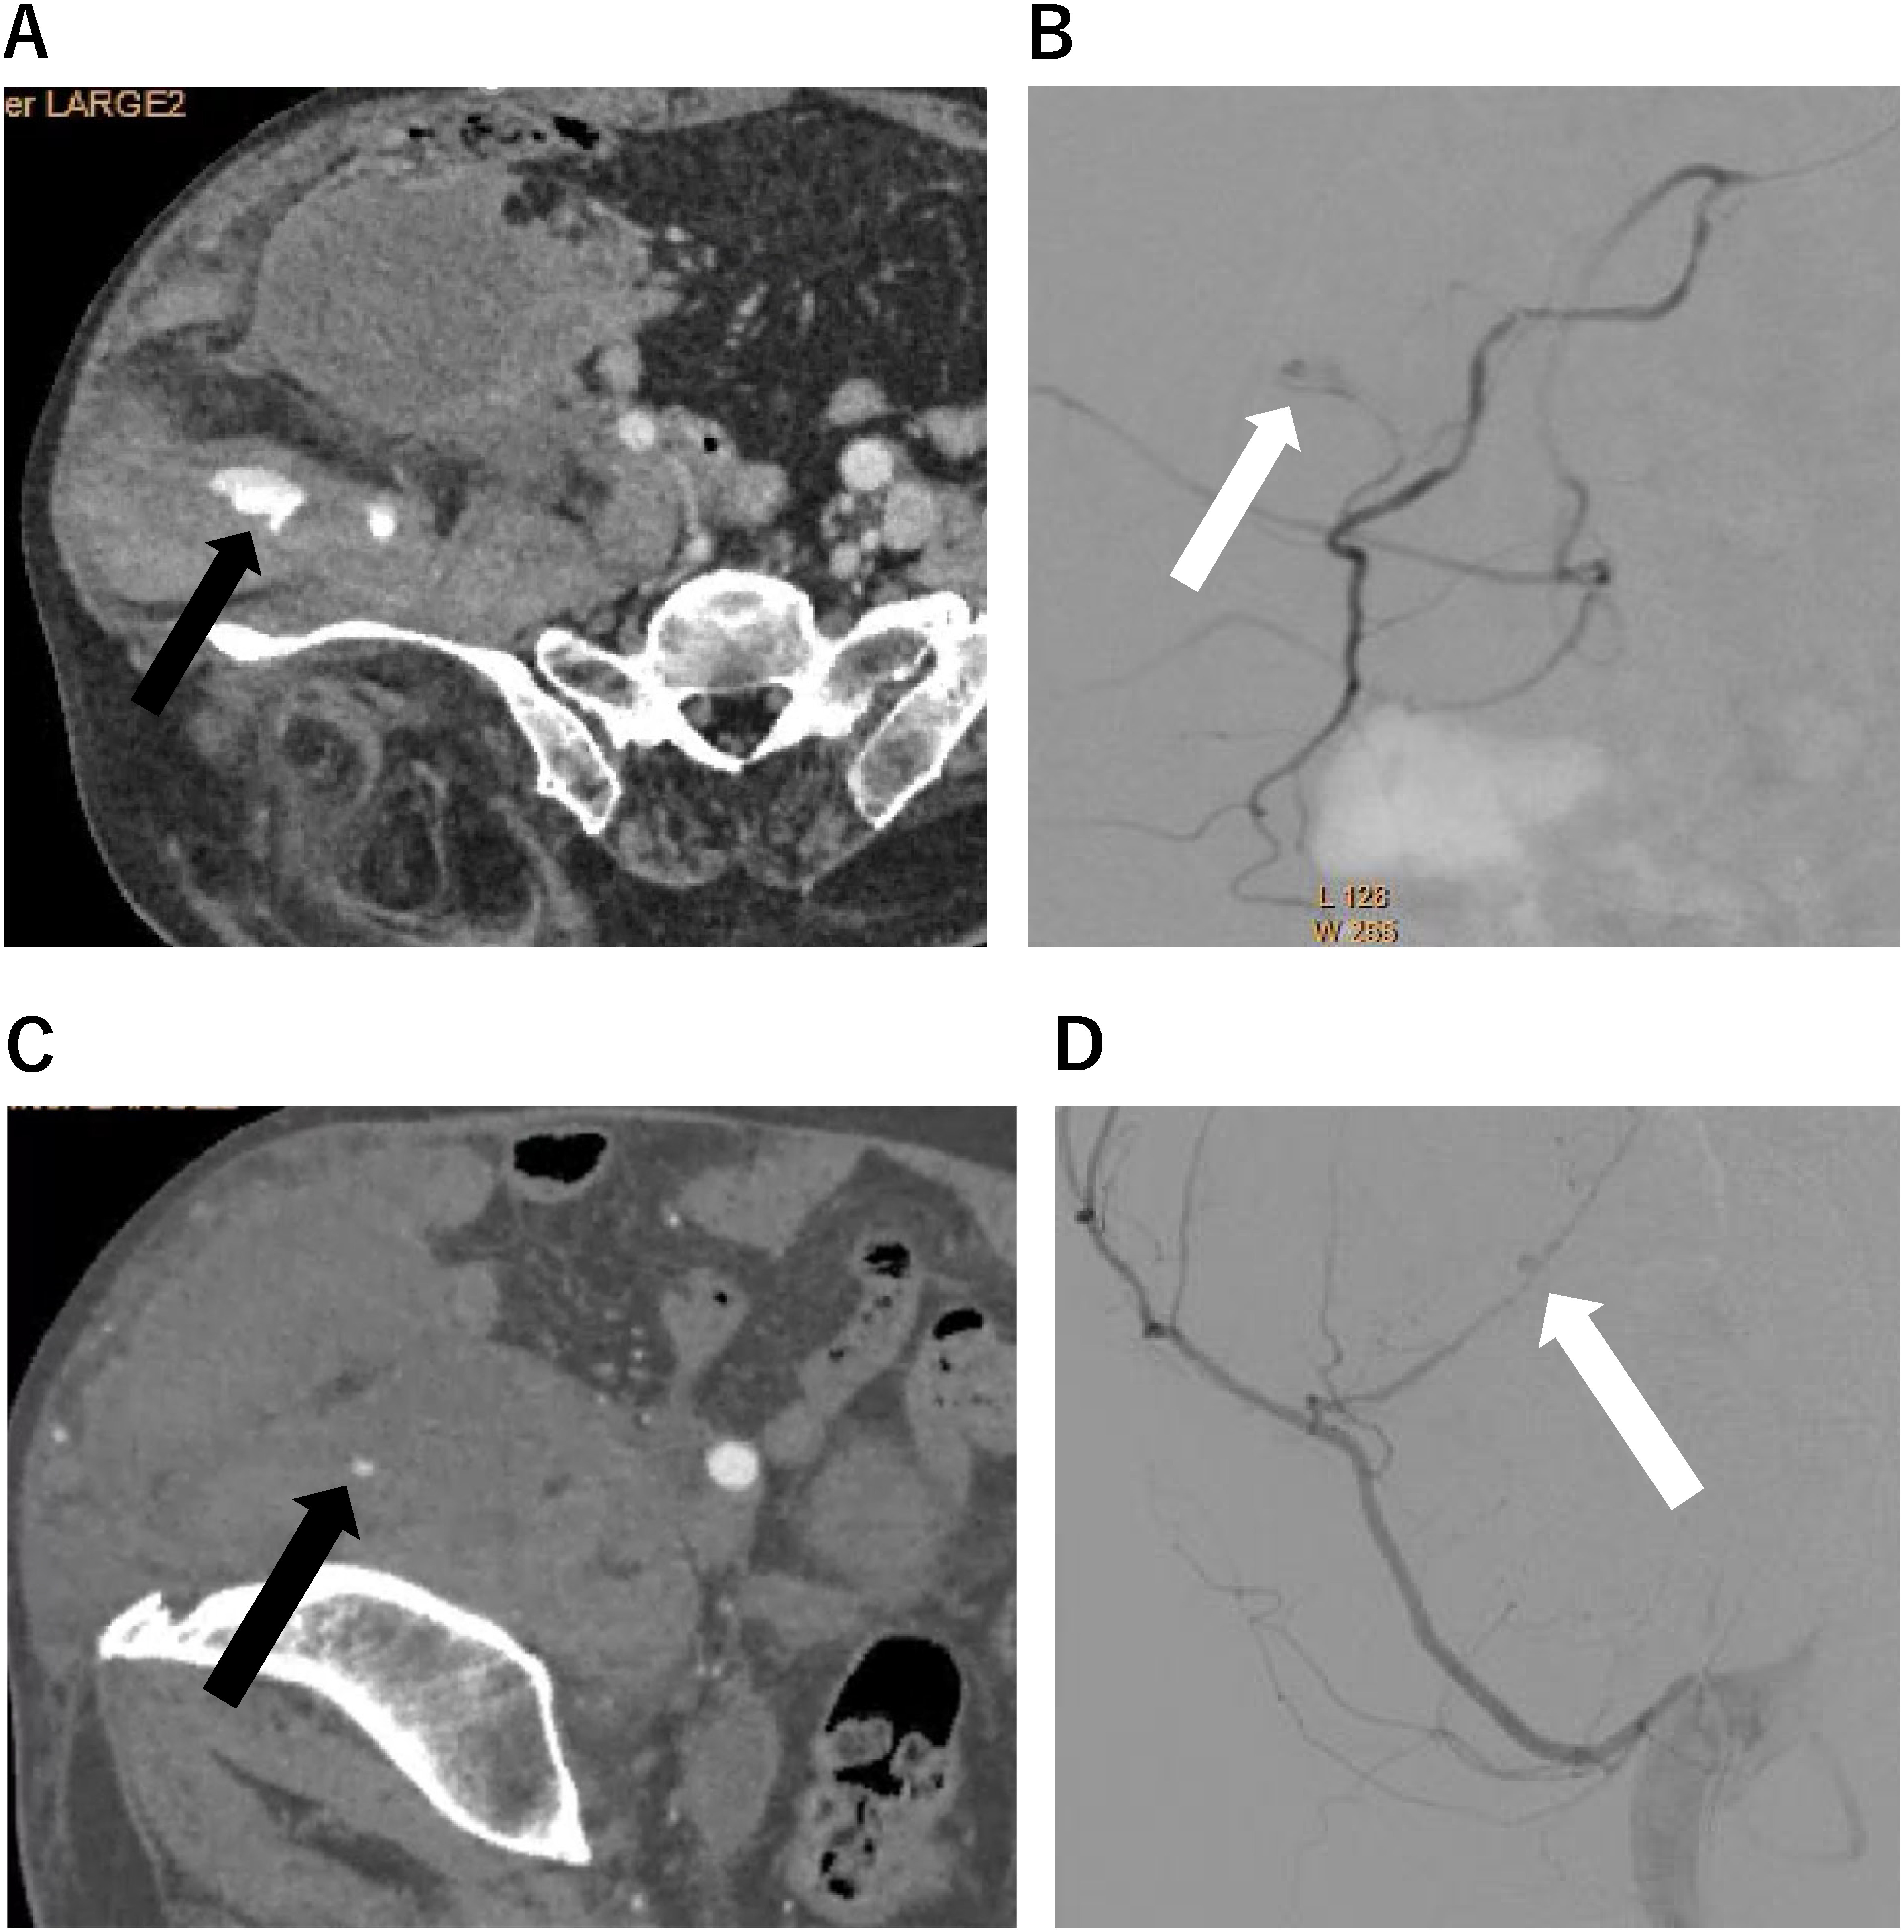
Fig. 3 Contrast-enhanced computed tomography (CT) and angiography on postoperative days 11 and 24. (A) Contrast-enhanced CT on postoperative day 11 shows a retroperitoneal hematoma and extravasation from the lumbar artery (black arrow). (B) An emergency angiogram shows extravasation from the lumbar artery (white arrow). (C) Contrast-enhanced CT at postoperative day 24 shows a pseudoaneurysm at the branch of the deep iliac artery (black arrow). (D) An emergency angiogram shows a pseudoaneurysm at the branch of the deep iliac artery (white arrow).
